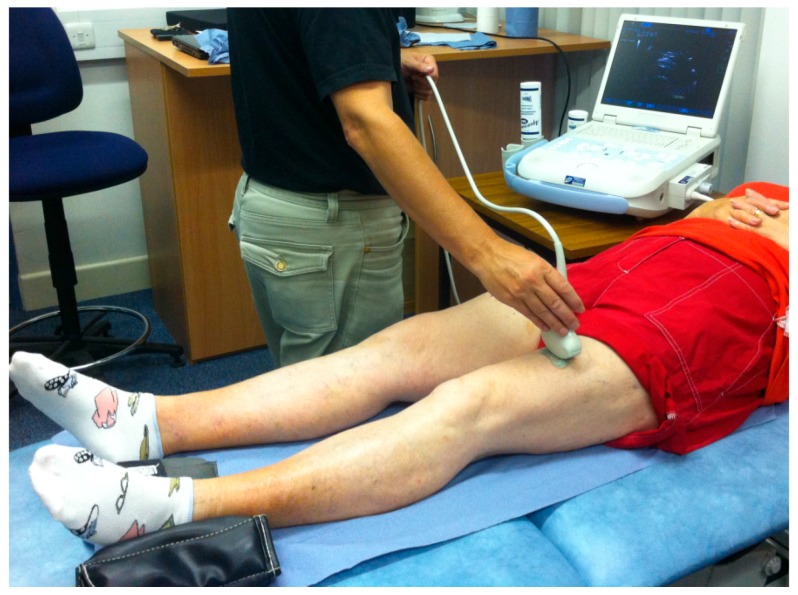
Ultrasound imaging set up with the participant in supine.
